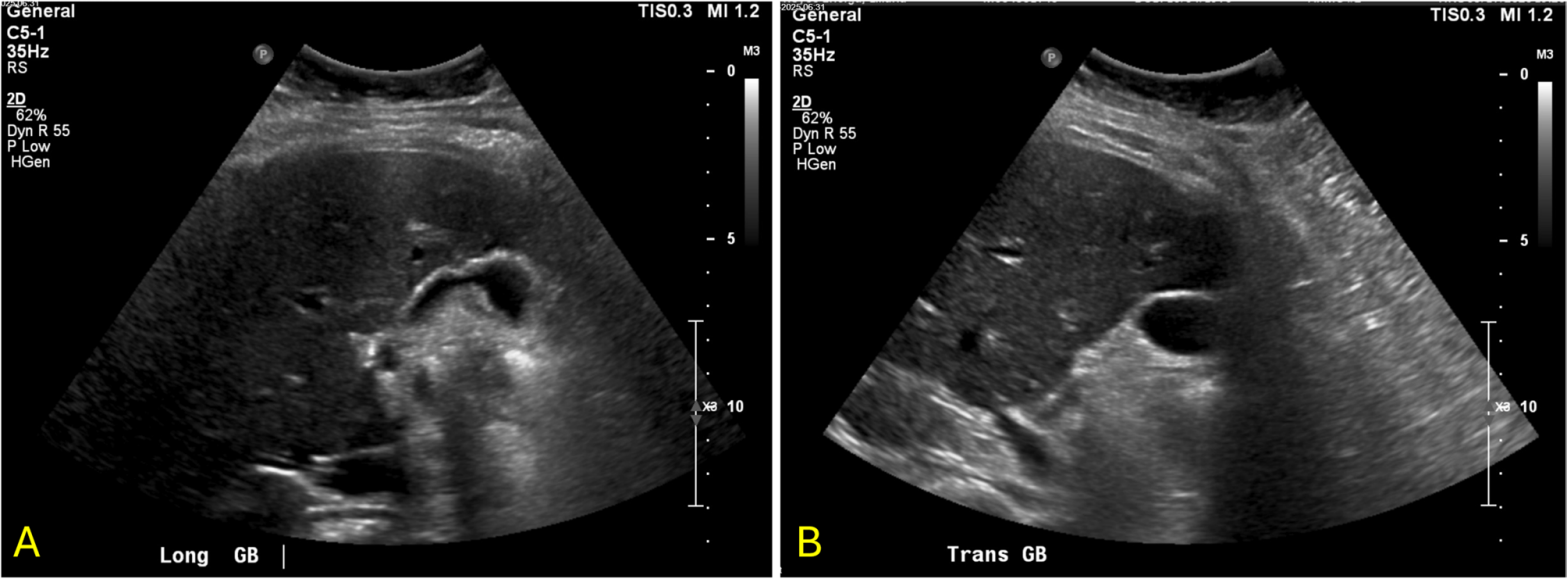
Ultrasound of the gallbladder and surrounding liver. A Longitudinal view and (B) transverse view demonstrating gallbladder without echogenic shadowing or wall thickening, and homogenous liver parenchyma with no clearly visible liver mass
